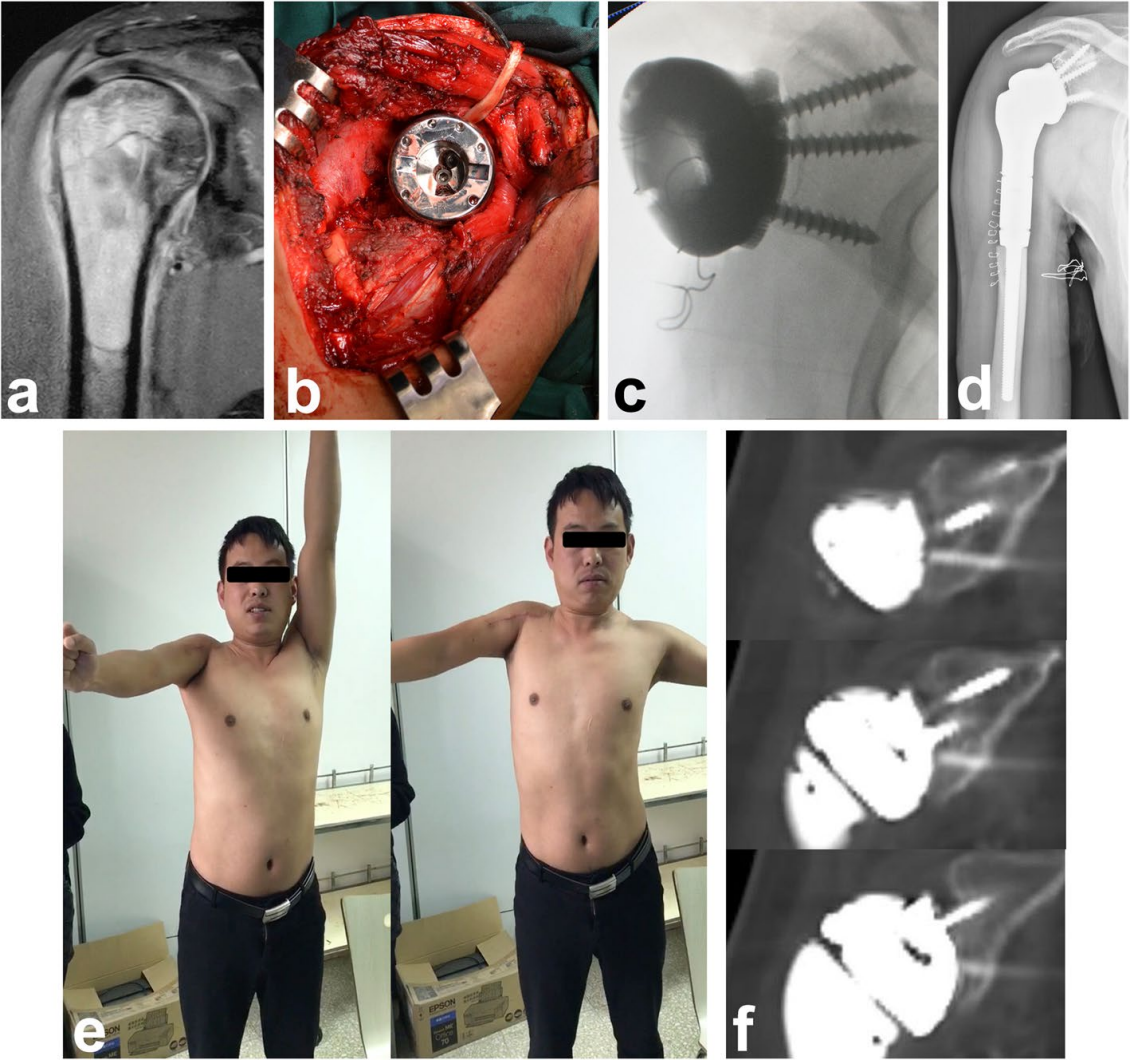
Application of the arthrodesis prosthesis. a A 30-year-old man diagnosed with malignant myoepithelioma in the right proximal humerus (patient #2). b The glenoid prosthesis was fixed to the scapula by three screws. c Intraoperative fluoroscopy showed good fixation of the prosthesis. d Postoperative X-ray showed good fixation of the prosthesis. e The patient showed satisfactory functional status of the right shoulder 3 months postoperatively with an MSTS-93 score of 27 and an ASES score of 81.7. f CT scan 12 months after surgery showed bone formation around the porous interface of the glenoid component
